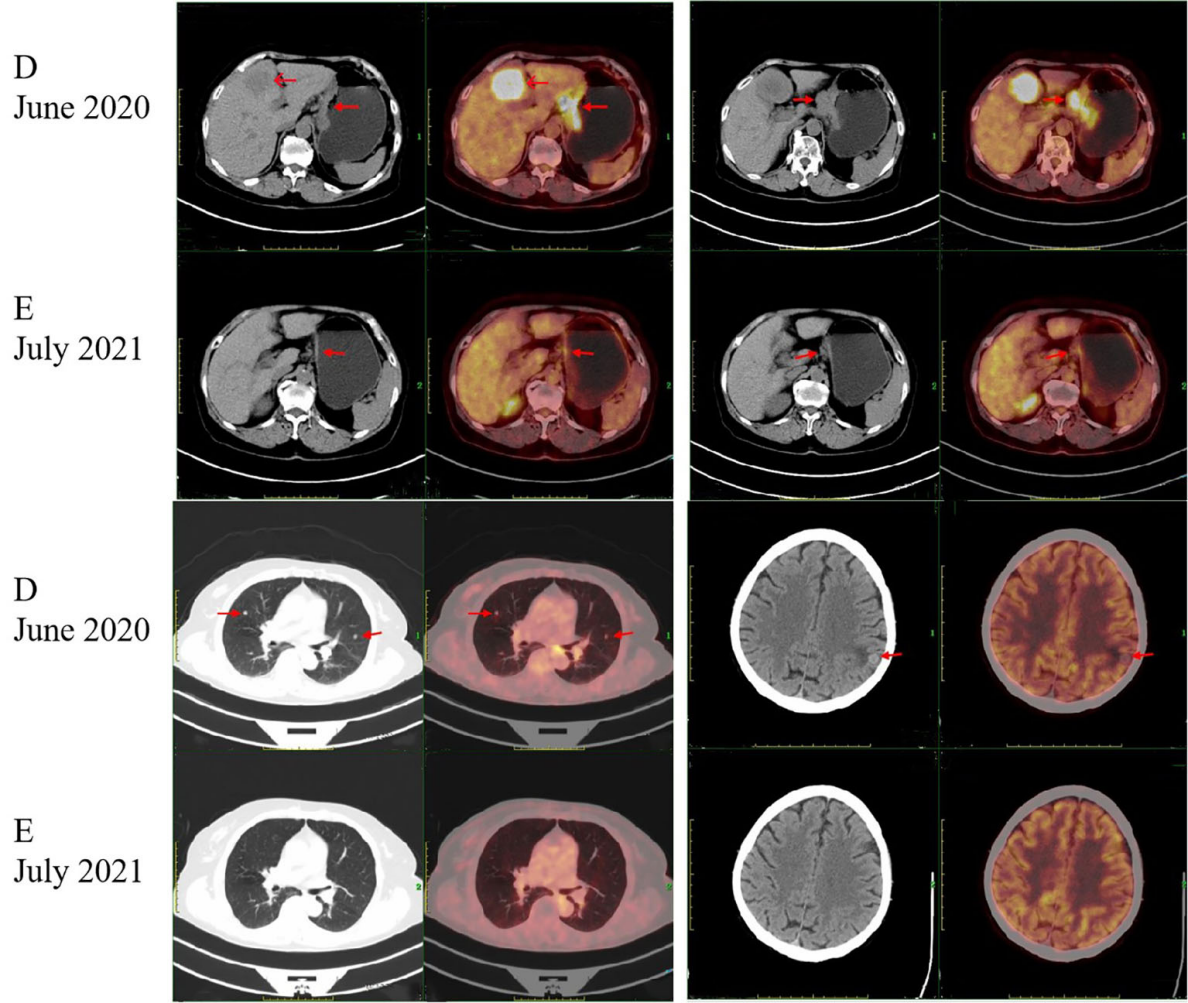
Positron emission tomography/computed tomography (PET/CT) images. Arrows indicate tumors. (D) PET/CT scan showed intense accumulation of the (18) F-fluorodeoxyglucose (FDG) metabolism at the cardia and the lesser curvature of the stomach, the gastric lesser omental lymph nodes, both lungs, liver and left parietal occipital lobe at the time of initial diagnosis. (E) After the HYPERLINK "javascript:;" treatment of pembrolizumab plus chemotherapy and pembrolizumab monotherapy, PET/CT scan showed that the lesions in the cardia and the lesser curvature of the stomach and the gastric lesser omental lymph nodes were significantly reduced and the metabolic activity was reduced. The lesions in both lungs, liver and left parietal occipital lobe disappeared.
